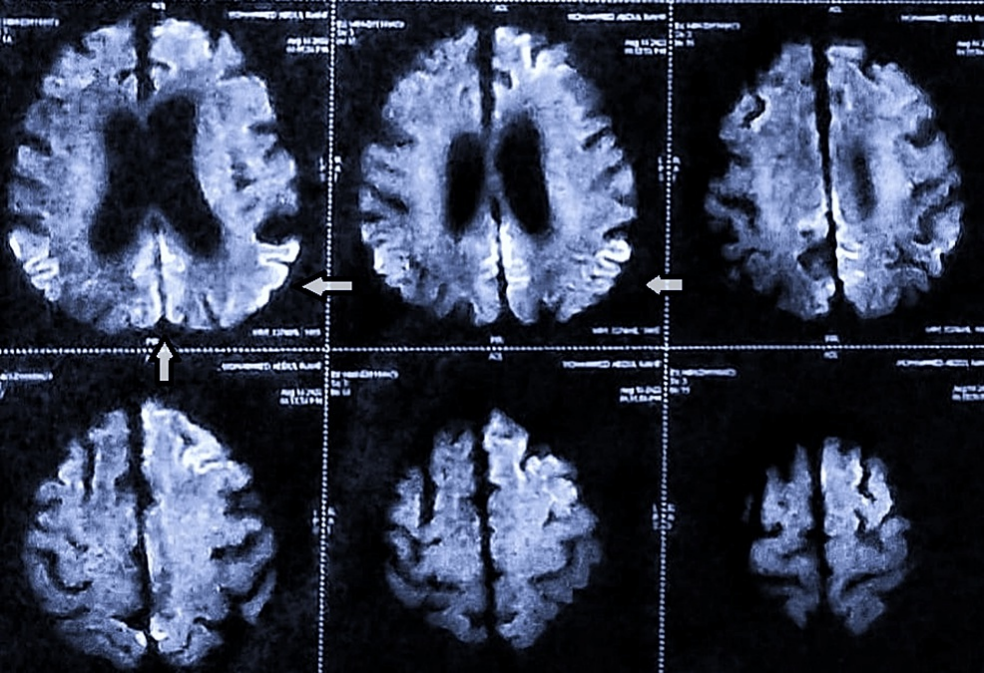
Diffusion-weighted MRI showing diffuse restriction of diffusion in the bilateral frontotemporoparietal regions.
